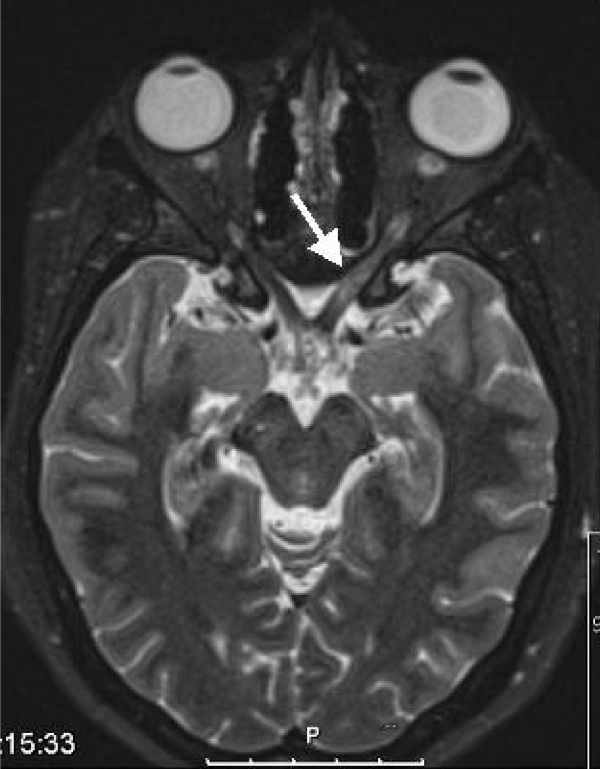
T2 weighted magnetic resonance imaging (MRI) image of the orbits. Nine days after the onset of visual loss, MRI shows slight swelling of the left optic nerve just proximal to the chiasm with mild signal changes also demonstrated.
